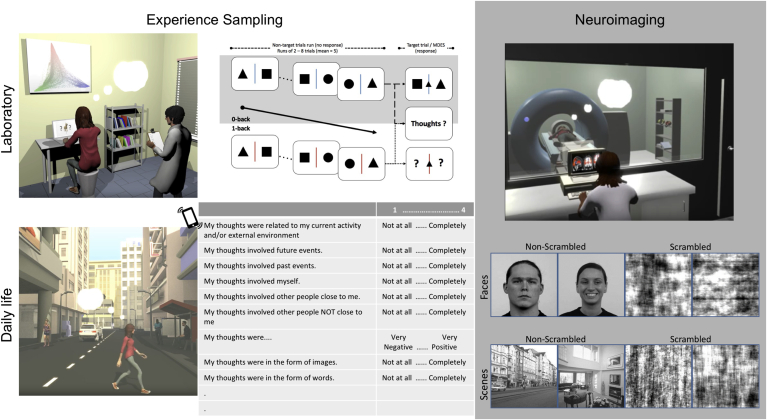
Study design. The study was designed to collect data from three different contexts: inside the MRI scanner, in the laboratory, and during daily life. Left and middle panel (white background): Experience sampling data was collected in two different contexts. (Top): In a laboratory context as participants performed a simple visual decision task with minimal semantic content. (Bottom): In a rich ecological context as participants went about their daily lives. In both contexts, participants described their ongoing experiences on multiple dimensions. Right panel (grey background): Structural and functional neuroimaging data were collected while participants were lying in the scanner passively viewing faces and scenes.
